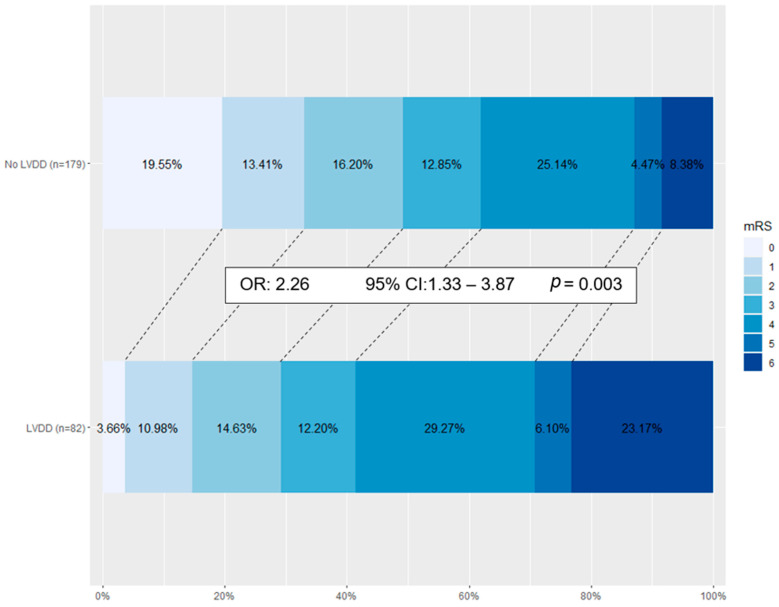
Ordinal shift regression analysis of 90-day modified Rankin Score comparing anterior large vessel occlusion stroke patients with normal and LVDD adjusted for age, gender, AF, LVEF, NIHSS, ASPECTS score, recanalization status, and time to reperfusion. AF, atrial fibrillation; ASPECTS, Alberta stroke programme early CT score; LVEF, left ventricular ejection fraction; NIHSS, National Institutes of Health Stroke Scale.
